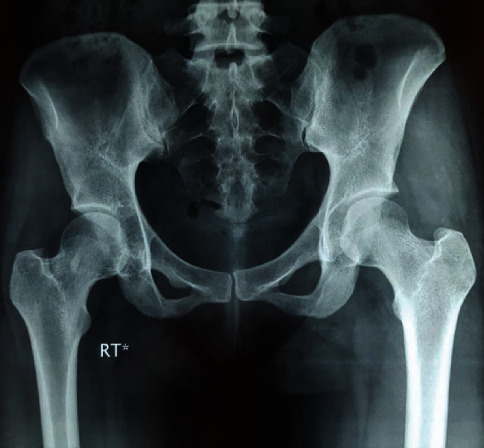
Preoperative anteroposterior pelvic X-ray of a 31-year-old female shows narrowing of joint space and cortical erosions and lucency in the acetabulum and femoral head of the right hip.
